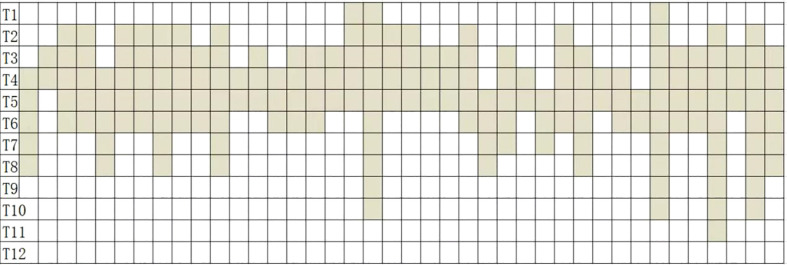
Skin hypoesthesia in 40 patients following ultrasound-guided TPVB.
